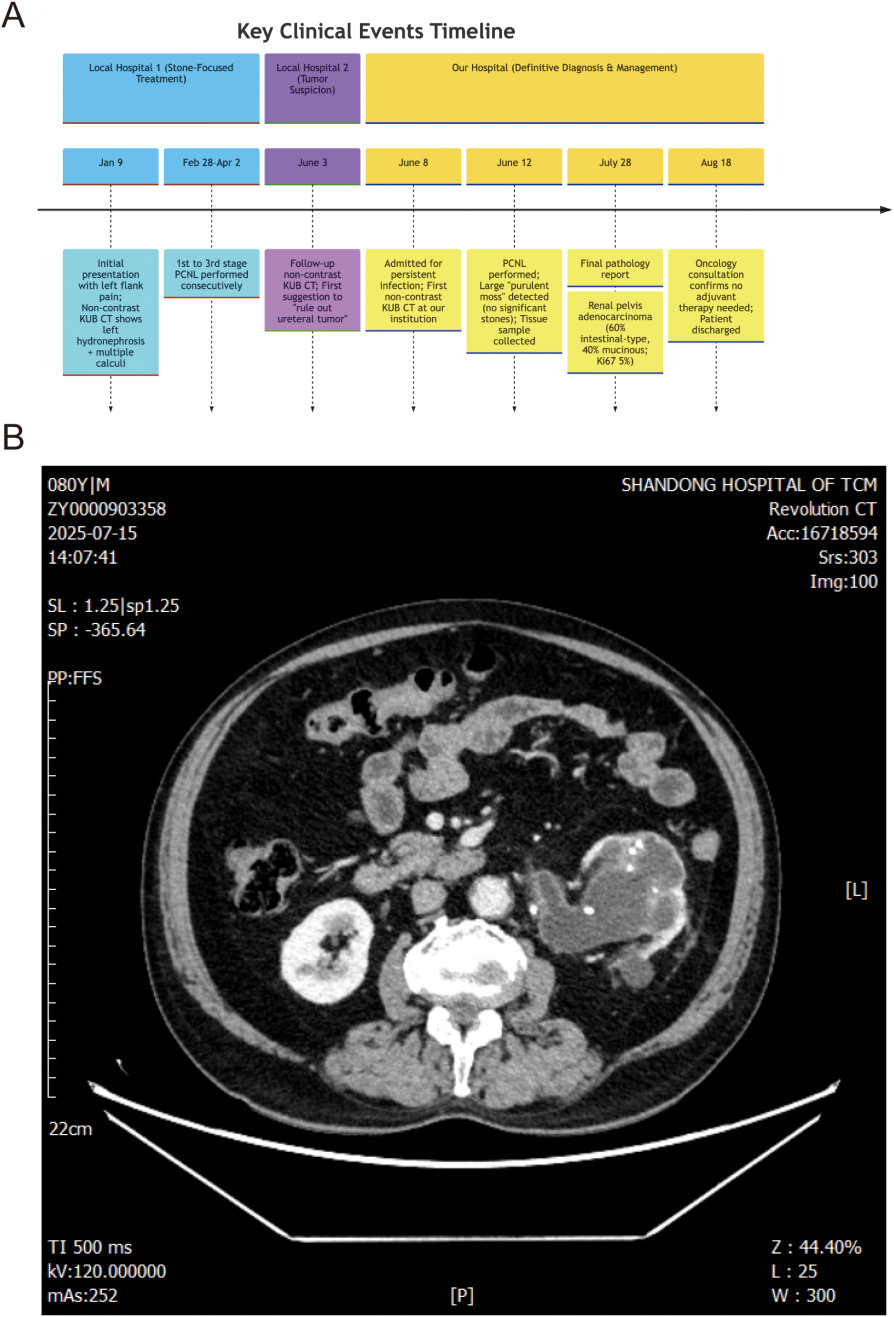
(A) Patient diagnosis and treatment route map. (B) Abdominal CT image demonstrating an enlarged kidney accompanied by multiple calculi.
